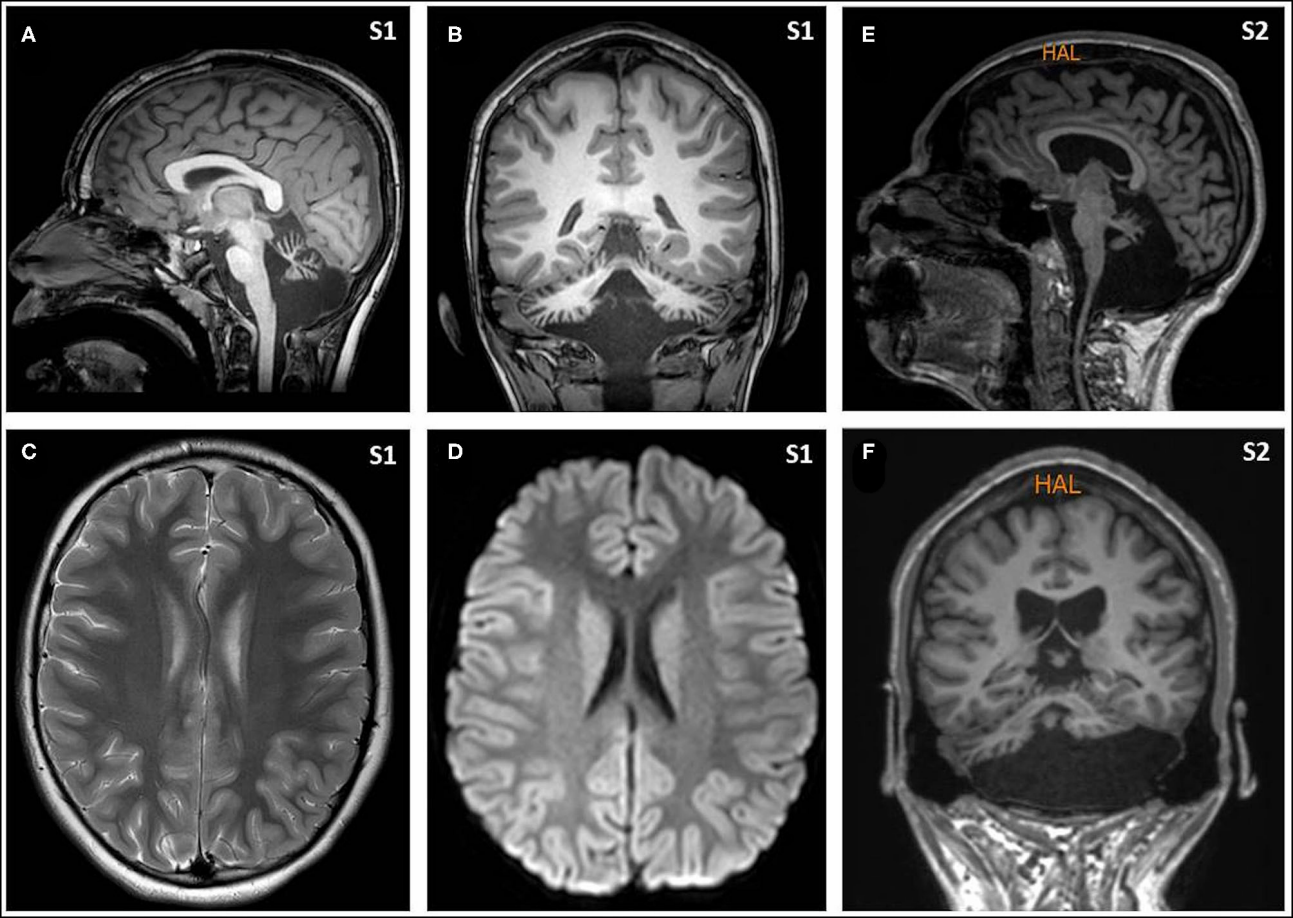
Magnetic resonance imaging (MRI) from Subjects included T1- and T2-weighted images and FLAIR, diffusion weighted images, and an arterial and venous angiographic study, performed with a 3 T and 1.5 T scanners, respectively. There was no abnormal finding other than the cerebellar atrophy. Sagittal [(A) from Subject 1, and (E) from Subject 2] and coronal images [(B) from Subject 1, and (F) from Subject 2] show the small cerebellar vermis with enlarged interfolial spaces representing atrophy and secondary enlargement of the fourth ventricle. Axial supratentorial images (C), including diffusion weighted images (D), from Subject 1 show no other abnormalities.
